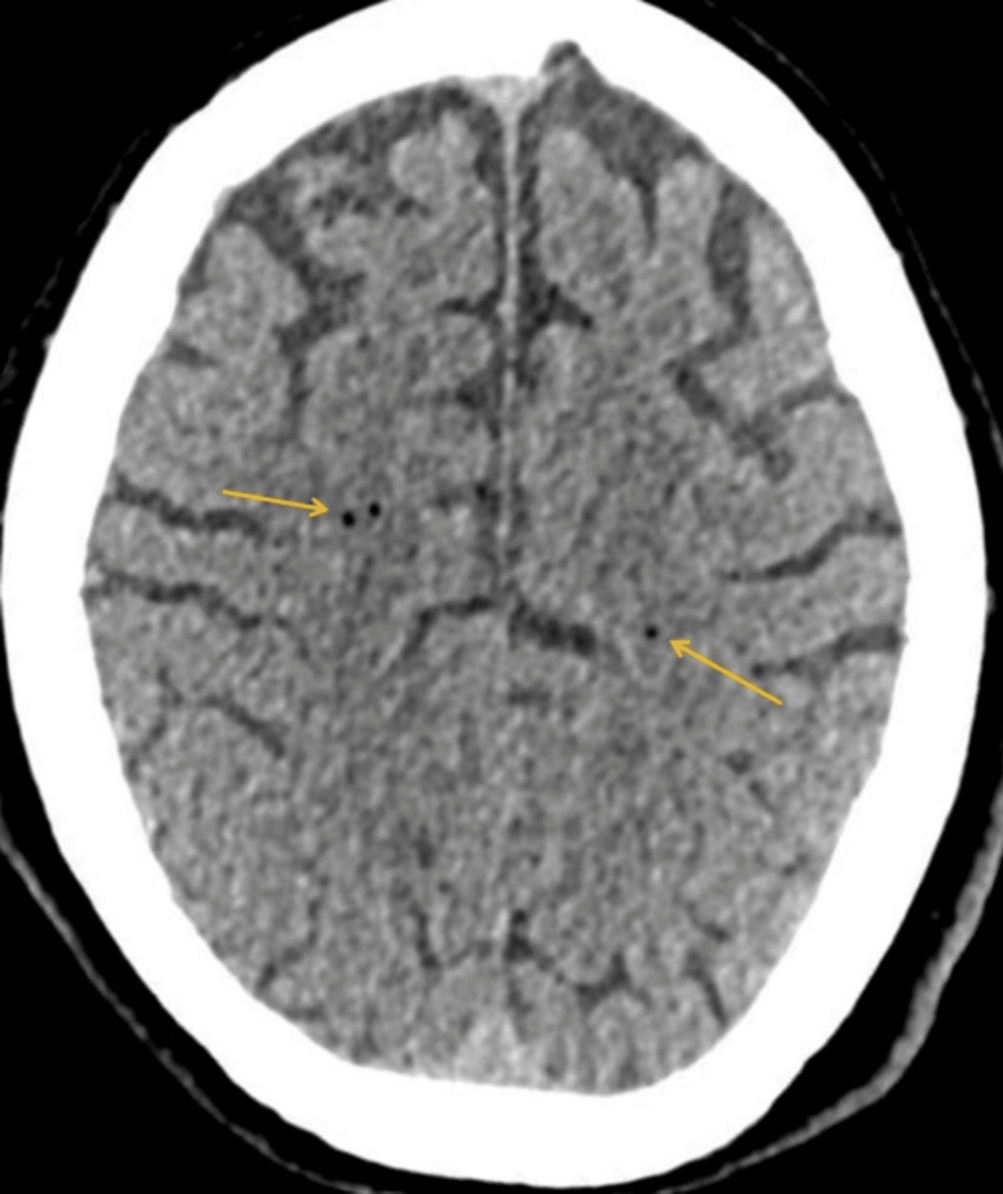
CT of the head axial non-contrast demonstrates a few small gas locules in the right centrum and the left semi-ovale in keeping with air emboli. CT: computed tomography
